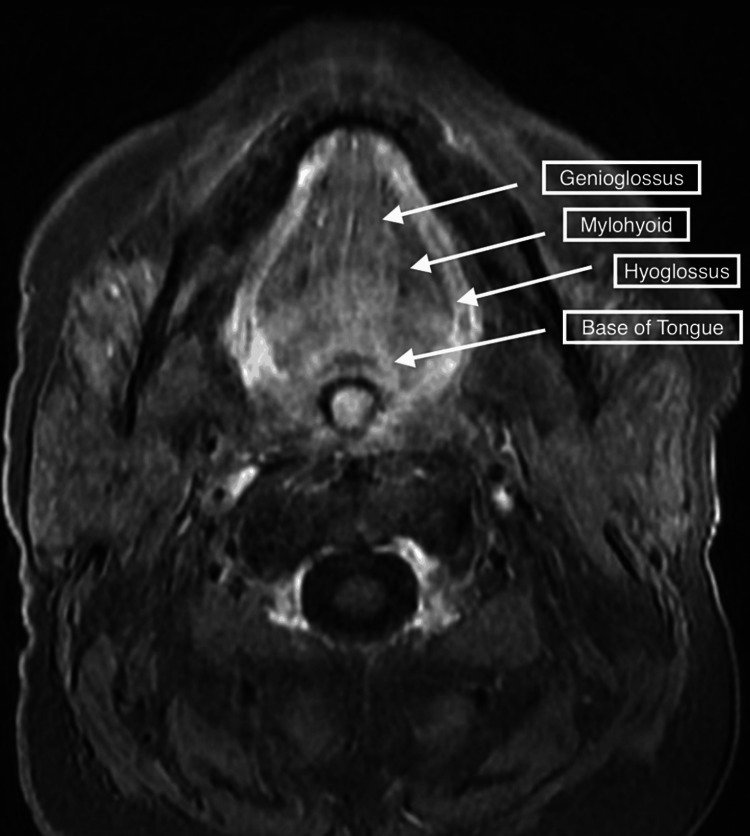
Axial gadolinium-enhanced T1-weighted image demonstrating tongue root and oral tongue muscular enhancement likely indicating acute myopathy or denervation.
